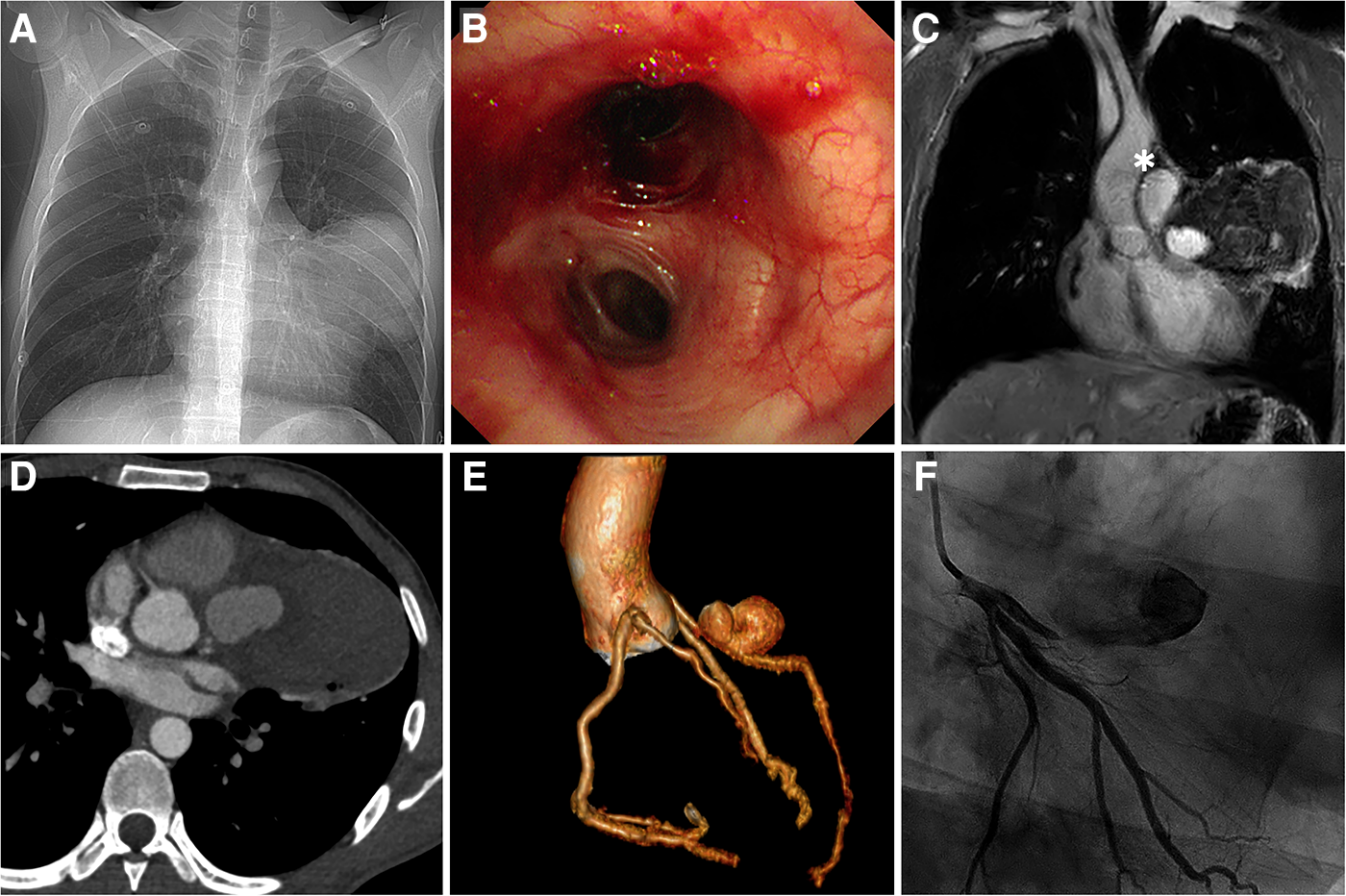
Multimodal imaging. (A) Anteroposterior chest x-ray. (B) Bronchoscopy view at the level of the left lobar bronchus. (C) Coronal view of thoracic MRI. (D) Coronary CT angiography demonstrating LAD with a large aneurysm wrapped in the hematoma (asterisk). (E) Preoperative coronary CT angiography: 3D reconstruction view. (F) Coronary angiography. LAD, left anterior descending artery.
